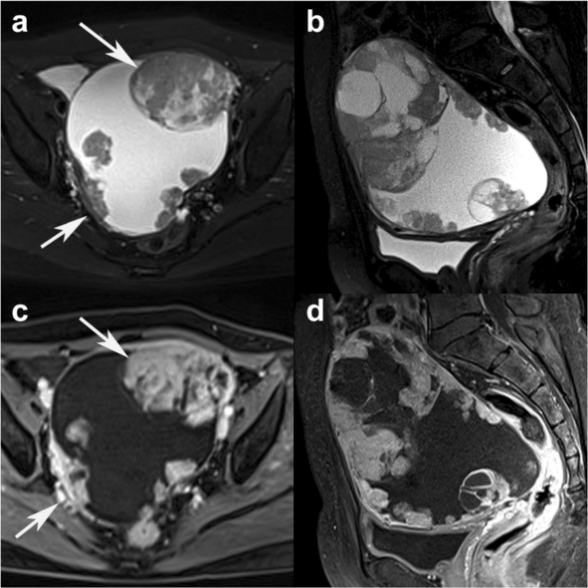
A 53-year-old woman with left ovarian clear cell carcinoma (CCC). Axial and sagittal turbo spin echo (TSE) T2-weighted imaging (T2WI) with fat saturation (FS) (a-b) show an oval unilocular cystic mass with papillary projections (arrows). Axial and sagittal contrast-enhanced flash 2D T1WI with FS (c-d) show prominent enhancements in solid components
